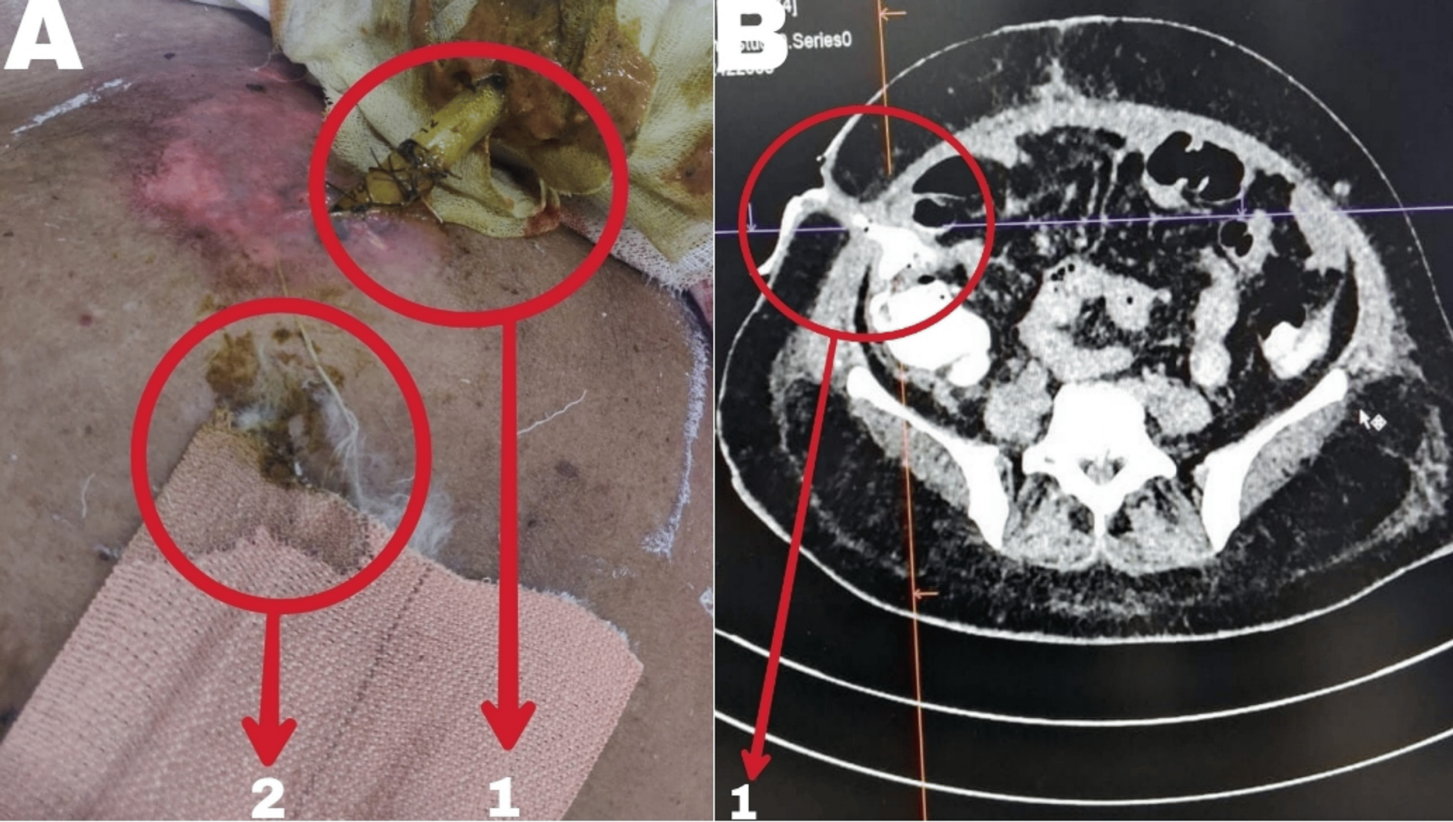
A) Fecal discharge from drain site and suture line on presentation; B) Contrast-enhanced computed tomography abdomen plus pelvis (CECT A+P) on presentation A1: fecal discharge from abdominal drain site with skin excoriation around drain site; A2: Fecal discharge from suture line with soaked dressing B: CECT (A+P) showing complete anastomotic dehiscence and contrast extravasation from ileal loops to drain site, suggestive of enterocutaneous fistula (5.5 mm thick, 35 mm long). Multiple intra-abdominal collections, indicative of peritonitis secondary to anastomotic leak B1: Enterocutaneous fistula (5.5 mm thick, 35 mm long)
